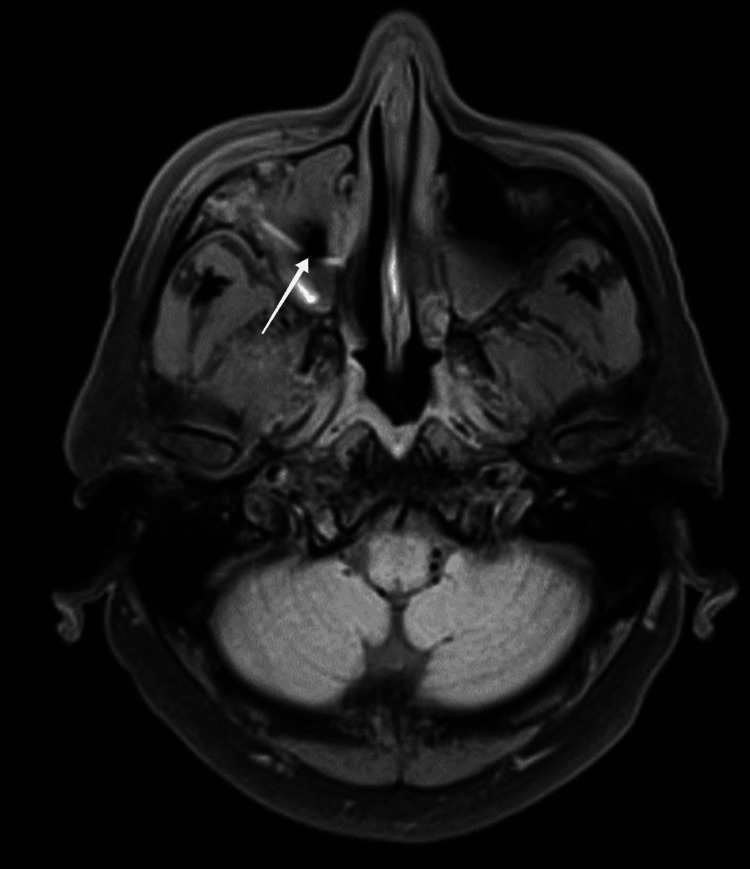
Axial T1-weighted pre-contrast image
